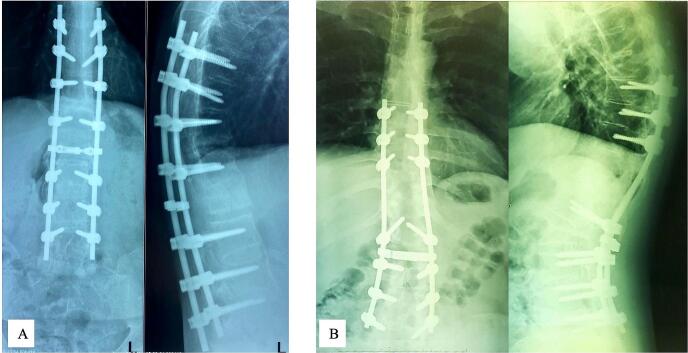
The last follow-up radiographic thoracolumbar X-ray evaluation of AP and lateral view in: (A) case 1 (24 months), (B) case 2 (36 months).
